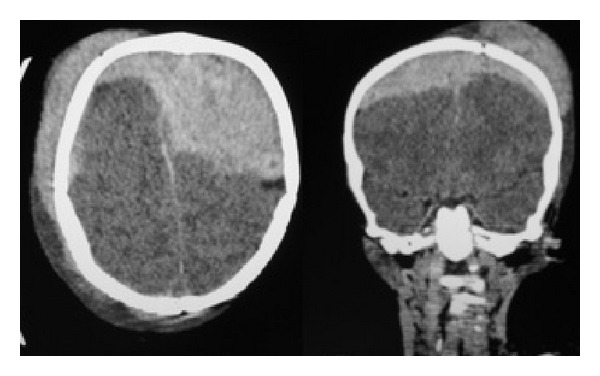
Axial and coronal CT scan images showing vertex epidural haematoma in preoperative course.
